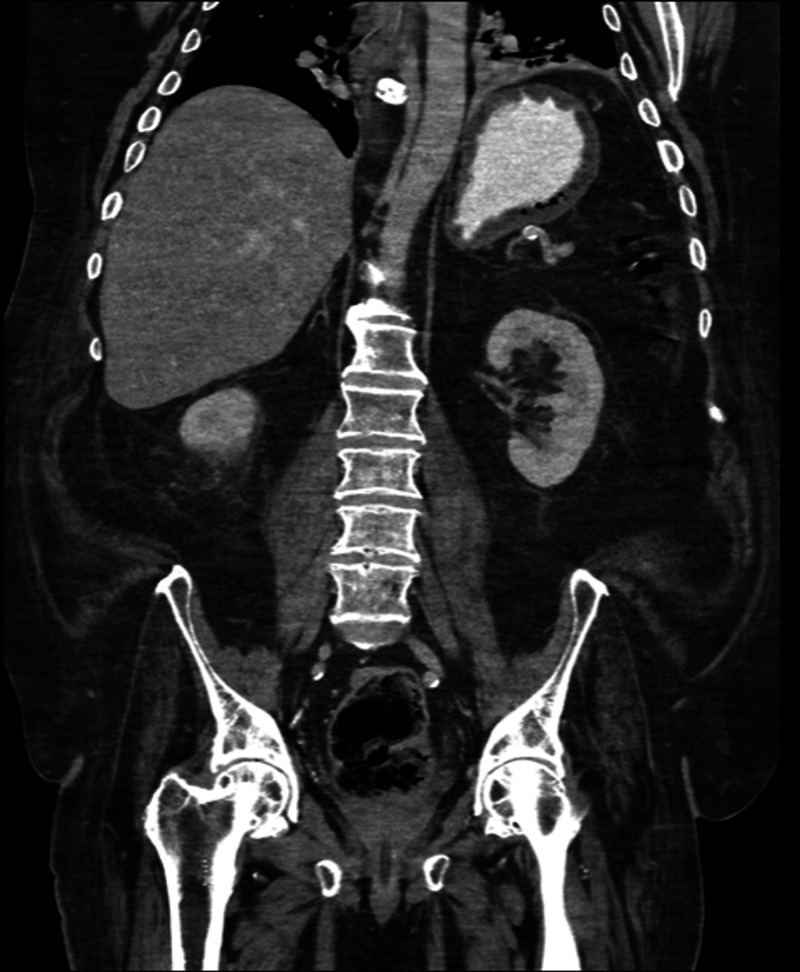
Coronal CT scan of the abdomen demonstrating resolution of air in the left gastric vein and the gastroepiploic veins. CT, computed tomography
